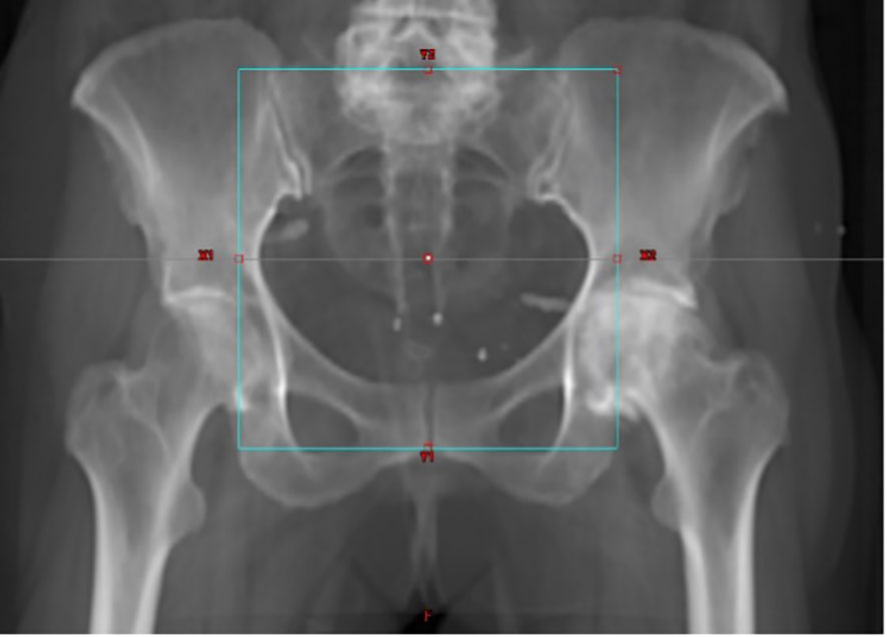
Digitally reconstructed radiograph in antero-posterior direction.
